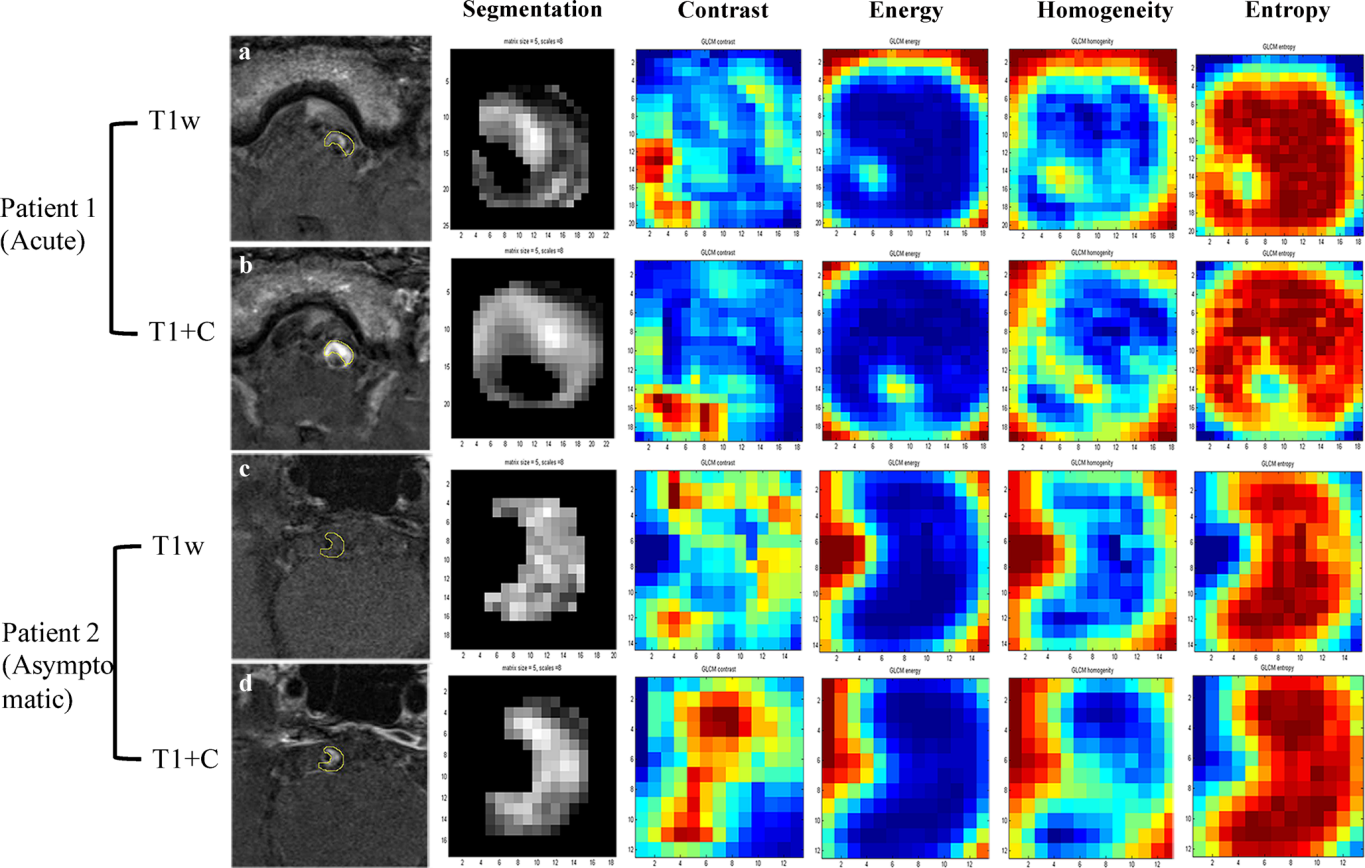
Radiomics analysis in two sample patients. Patient 1 is a 63-year-old man with acute stroke on the stem. Patient 2 is a 57-year-old man free of symptoms. T1-weighted images are shown in a and c, and CE-T1 images are shown in b and d. Four representative GLCM radiomics features from 94 features are shown (contrast, energy, homogeneity, entropy)
